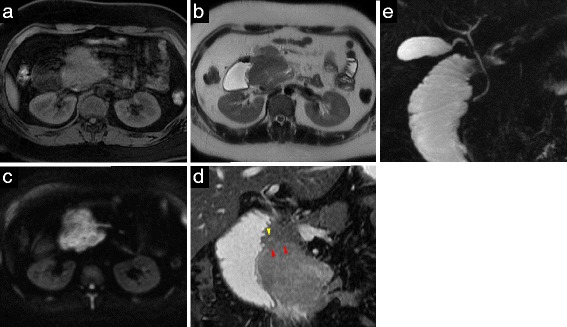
MRI of the abdomen. a T1-weighted images showed an isointense tumor compared with the pancreas. b T2-weighted images showed a slightly hyperintense homogenous tumor. c Diffusion-weighted images showed a hyperintense tumor. d FIESTA showed that the tumor edge (the *red arrow*) and the common bile duct in the pancreas (the *yellow arrow*) were located slightly distant. e MRCP showed that the common bile duct was not expanded and the main pancreatic duct was not depicted because it was too narrow
